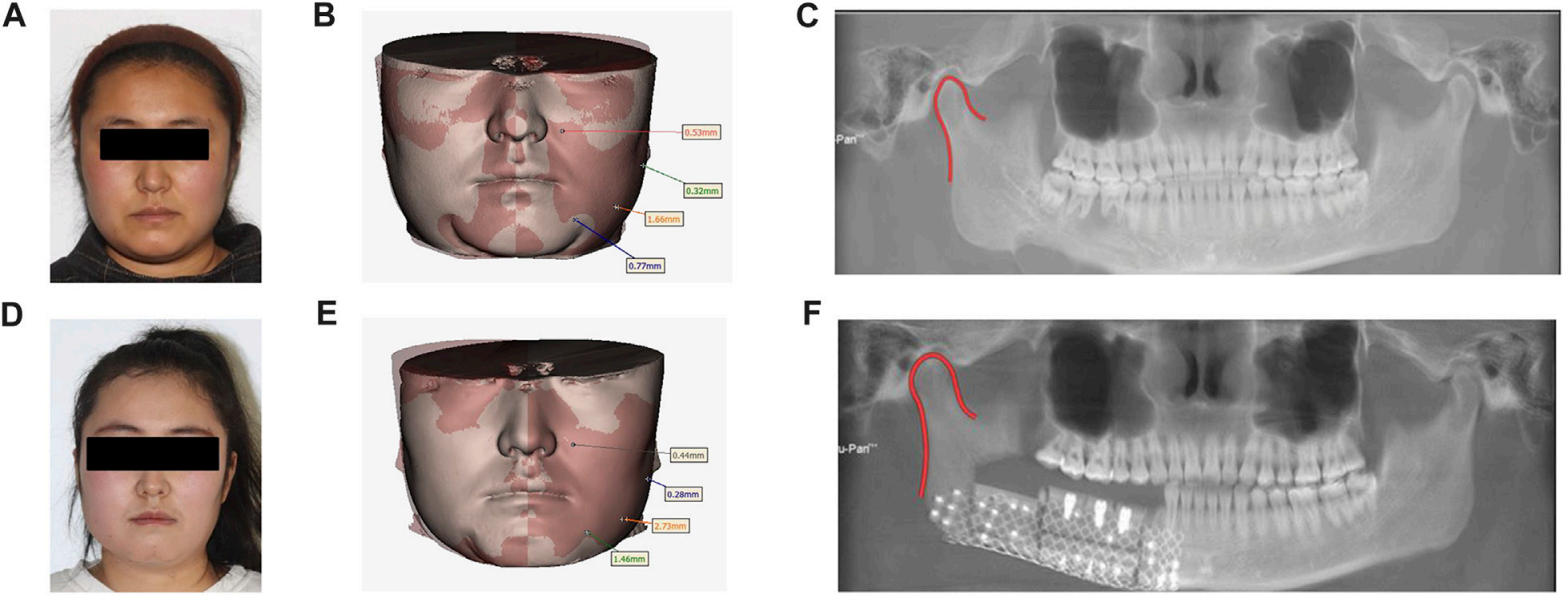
Preoperative and postoperative contrast: (A) Preoperative facial features. (B). Preoperative facial soft tissue mirror image fitting was performed. (C). Preoperative panoramic radiographs. (D). Postoperative facial features. (E). Postoperative facial soft tissue mirror fitting. (F). Postoperative panoramic radiographs.
